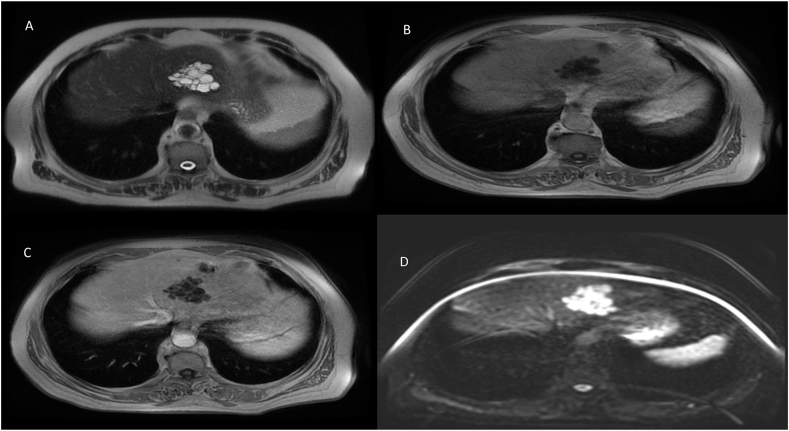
Image 2a: T2-weighted MRI axial: a well-defined multilocular intrahepatic mass is depicted, with high-intensity fluid content and multiple internal septa. 2b: T1-weighted unenhanced MRI axial: the lobulated intrahepatic mass has low heterogeneous signal intensity. 2c: T1-weighted MRI axial portal, delayed phase: the enhancement of the internal septa, is more obvious.2d: Diffusion-weighted MRI (ADC): the fluid in the cystic lesion show no restricted diffusion. There are thick internal septa.
